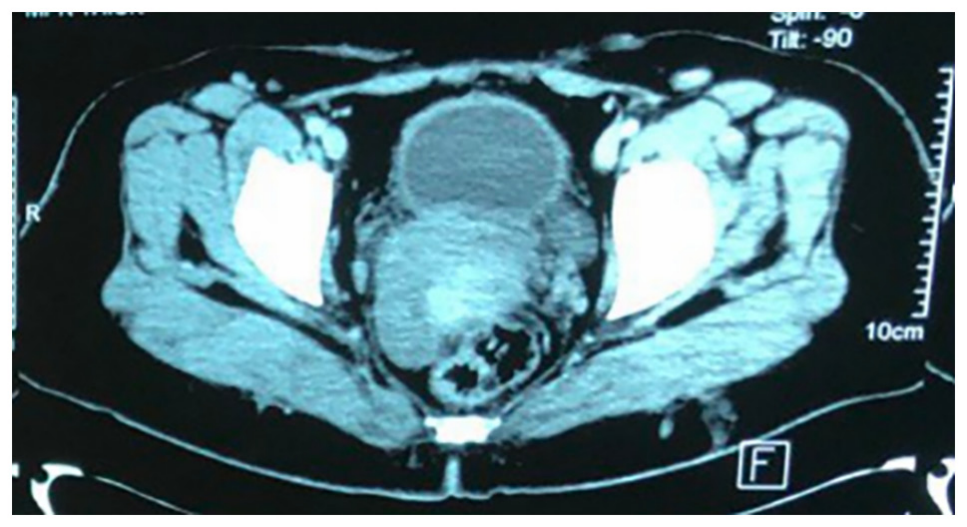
A contrast-enhanced CT scan of the pelvis indicating a mildly thickened urinary bladder wall; however, no obvious mass is present, and the bladder appears small in size.
